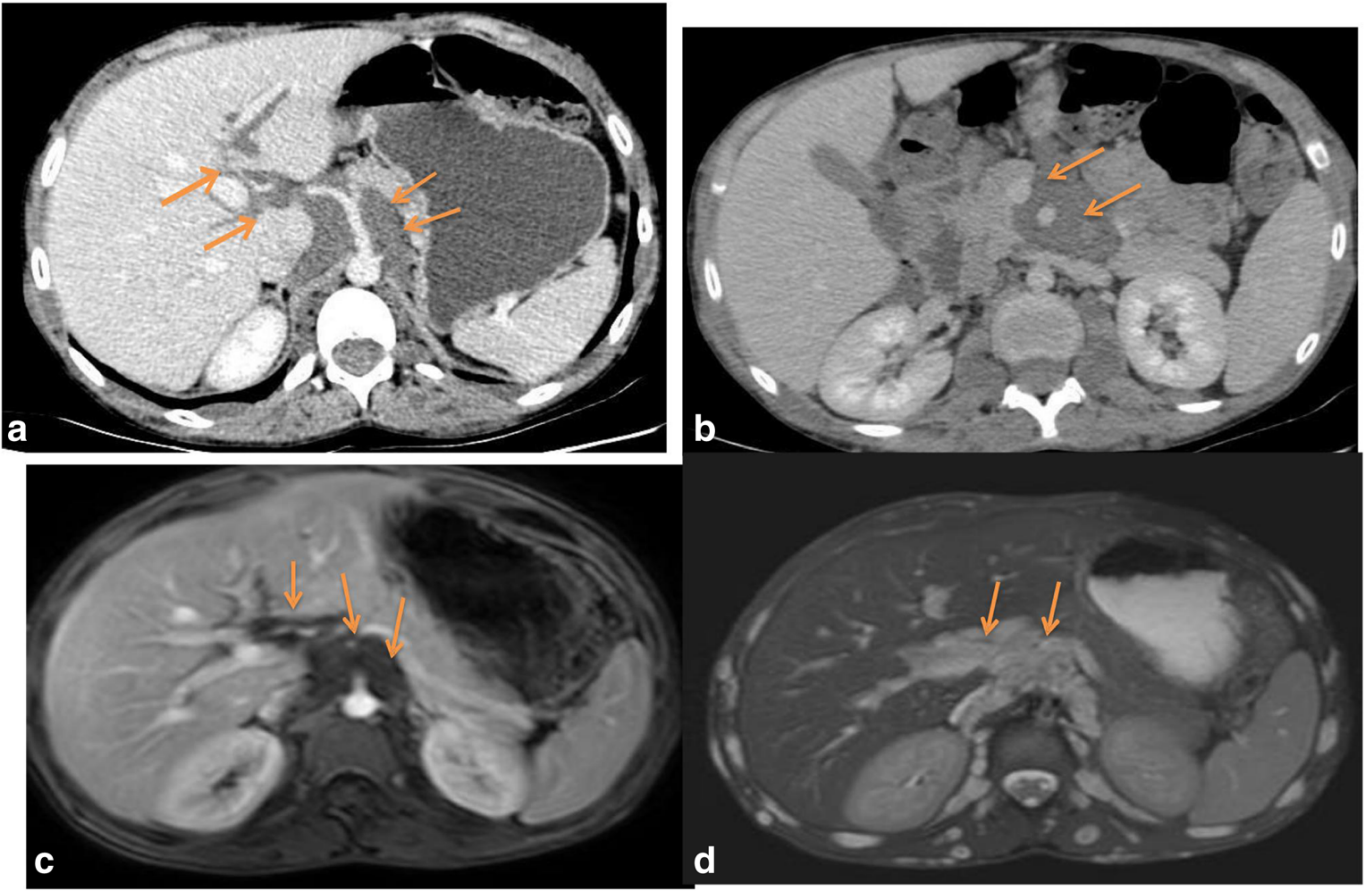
A 13-year-old boy with systemic NF1. Small bowel CT scan (a, b) and MRI (c, d) show a lobulated mass surrounding the retroperitoneal vessels extending from the left lateral aortic region to hepatic hilum (arrows)
